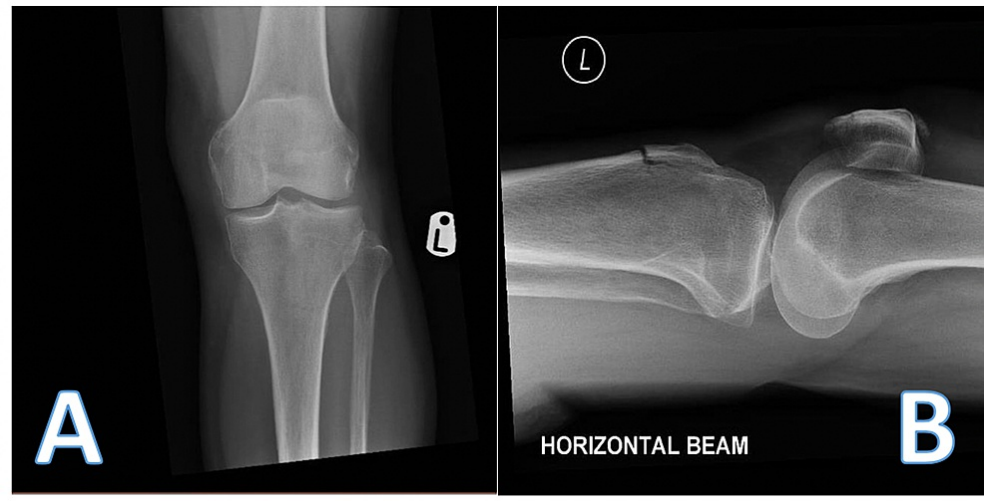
Pre-operative anterior-posterior (A) and lateral (B) radiographs taken in the emergency department.
